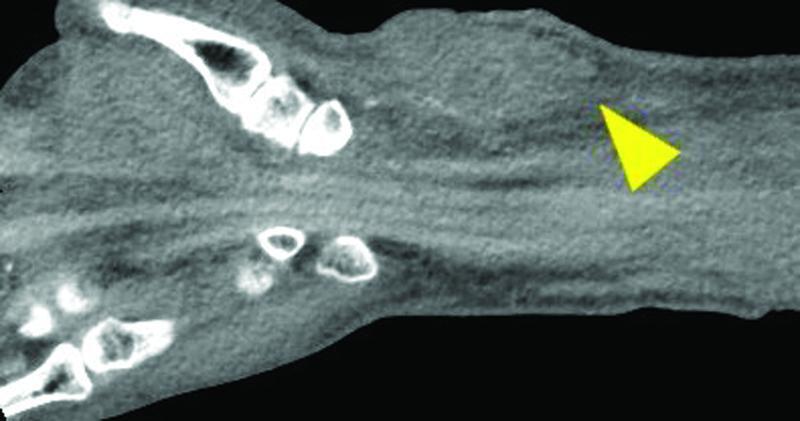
Clinical imaging (computed tomography [CT]): CT revealed low-echoic lesion at his left wrist, suggesting an infected pseudoaneurysm (yellow arrowhead).
